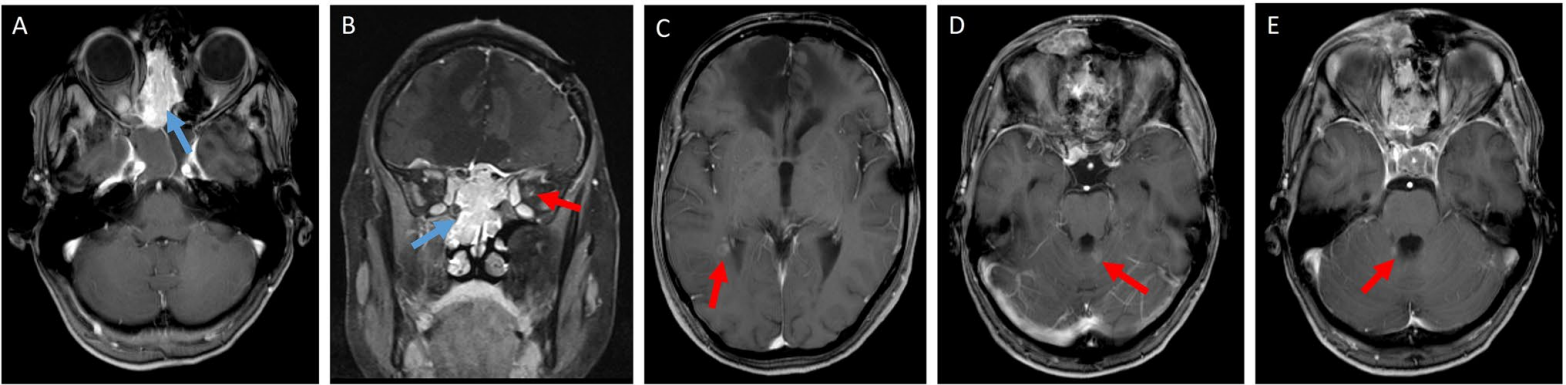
Equivocal clinical symptoms in concomitant MS and CNS tumor. This 46-year-old female patient has been diagnosed with multiple sclerosis and recurrent multifocal meningioma. The latter has been resected surgically twice and treated with a radiation therapy after the last detection of tumor growth. Three months after the radiation therapy the patient presented again with a vision loss in the left eye. Optic nerve compression by a new tumor growth was suspected. However, the patient reported also new general weakness, numbness in all four extremities, unsteady gait and clinically a sixth nerve palsy on the right side was detected. Brain MRI did not show any new tumor growth (A–B, blue arrows) but revealed several new gadolinium-enhancing MS lesions including one in the optic nerve (C–E, red arrows). Corticosteroids resulted in improvement of all symptoms, but the vision loss progressed further. Plasmapheresis was initiated and resulted in a partial improvement of vision after 9 cycles. Radiogenic damage to the optic nerve was also considered as an alternative diagnosis but the effect of plasmapheresis suggested inflammatory MS activity
